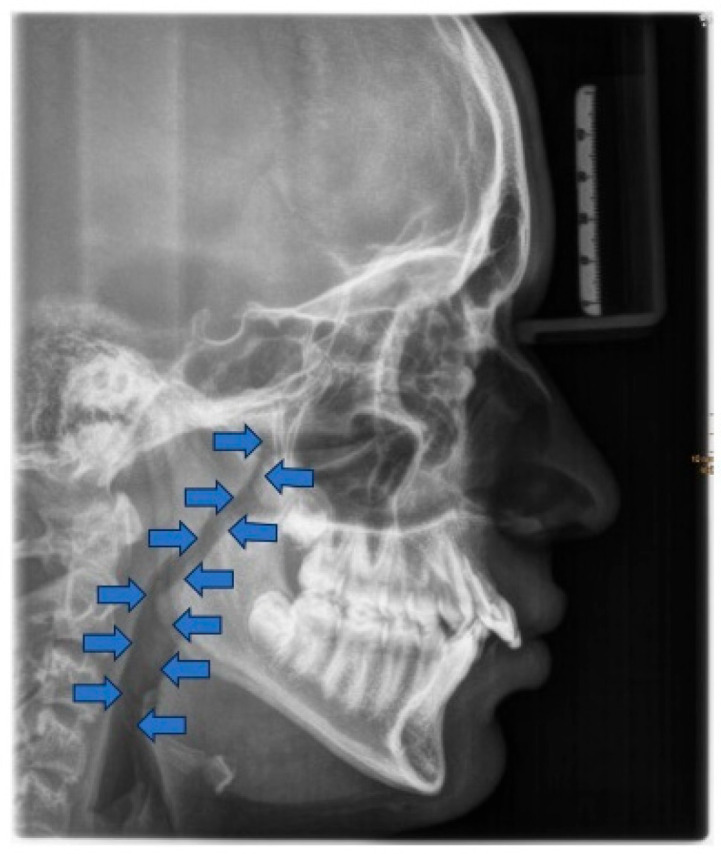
Radiographic cephalogram: Blue arrows indicate the posterior airway space.
